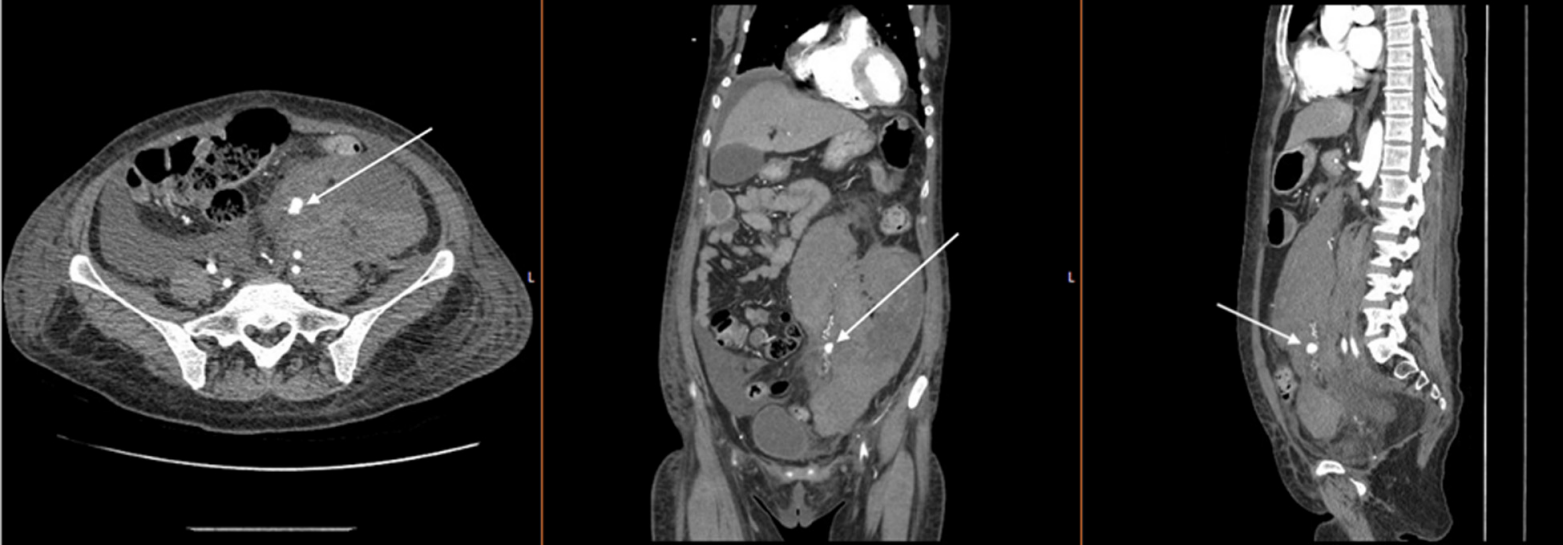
Computed tomography angiography demonstrating extravasation from left ovarian artery aneurysm (arrows).
